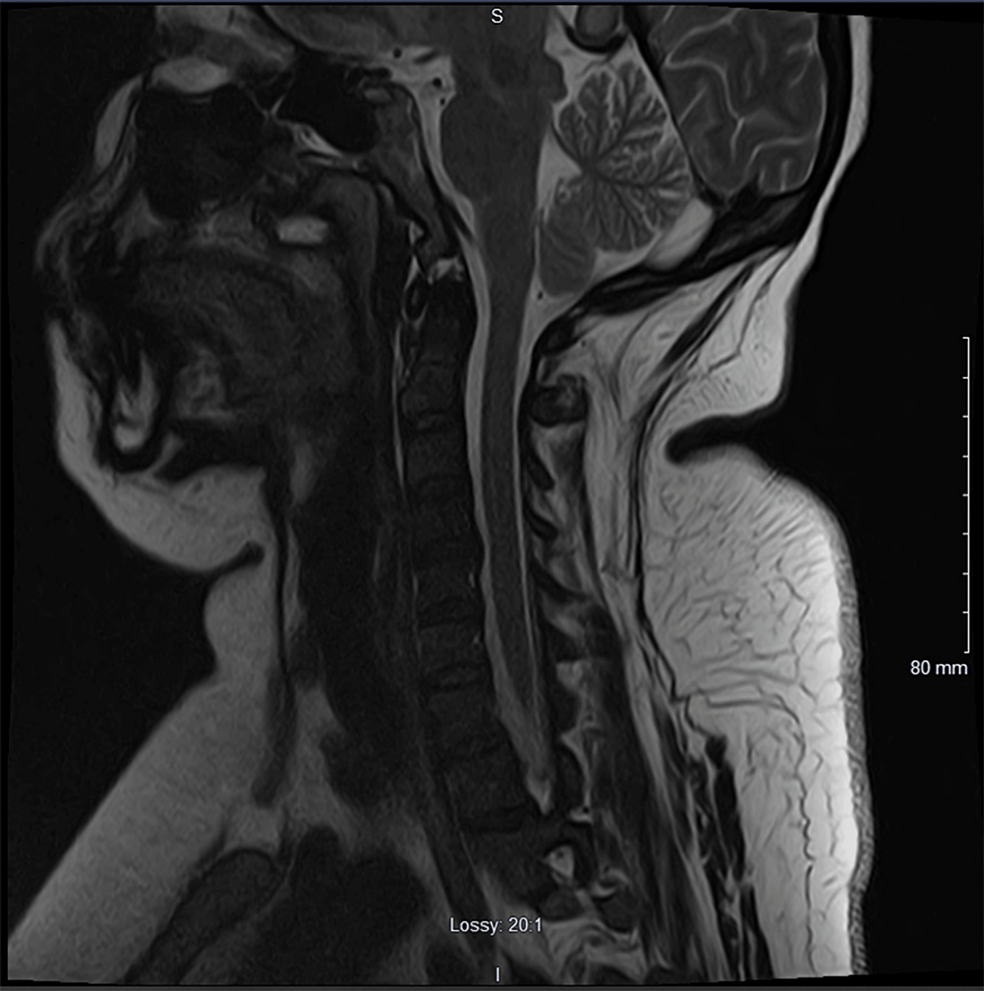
Unremarkable MRI cervical spine.
